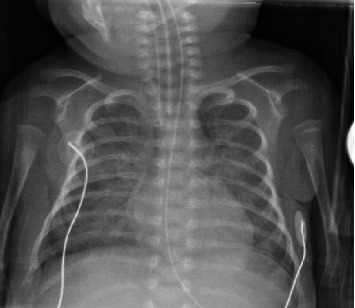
AP chest x-ray initially revealing foreign body within the left mainstem bronchus.
